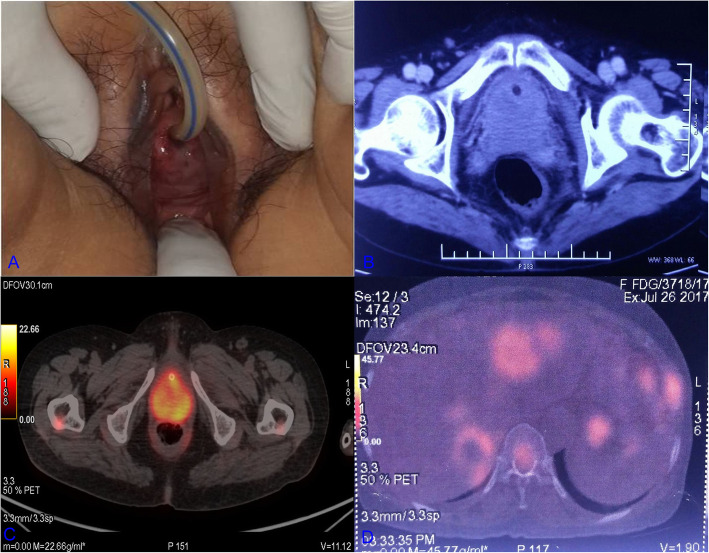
Clinicoimaging findings: (a) Local examination revealed a mass with bluish tinge stretching the external urethral meatus. A catheter was placed which drained clear urine. (b) Computed tomography scan showed a large urethral mass which was further highlighted with (c) positron emission tomography scan. (d) In addition, multiple metastatic lesions were seen in the liver
